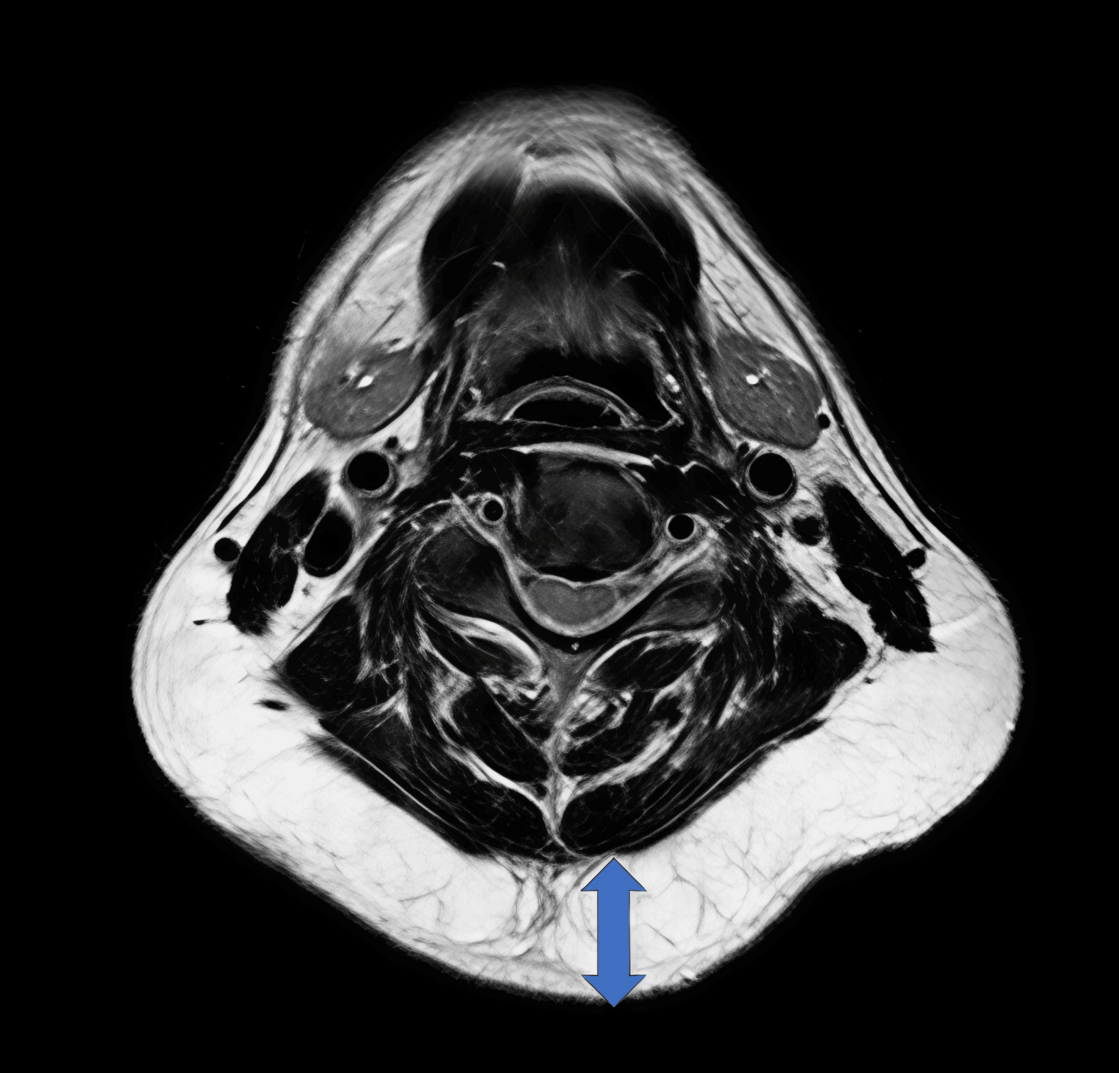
Initial MRI imaging of cervical benign symmetric lipomatosis T1-weighted axial image showing symmetrical, non-encapsulated adipose proliferation measuring 21 mm at the C4/5 vertebral level, with predominance in the posterior cervical region.
